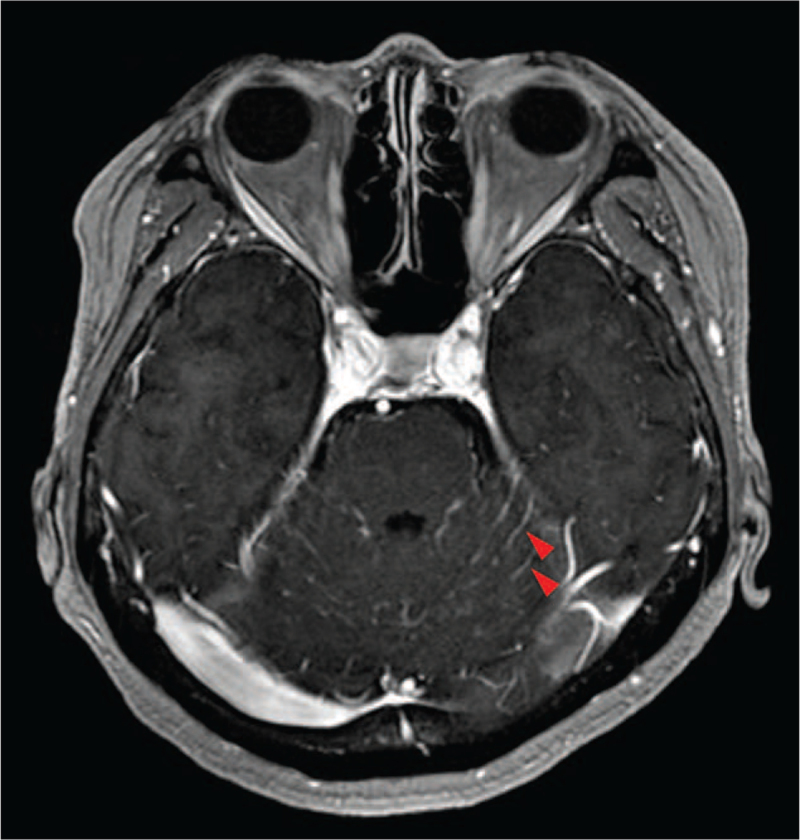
Brain contrast-enhanced magnetic resonance imaging when a disturbance of consciousness and diplopia occurred, shows diffuse, and linear enhancement along the cerebellar folia (arrows).
